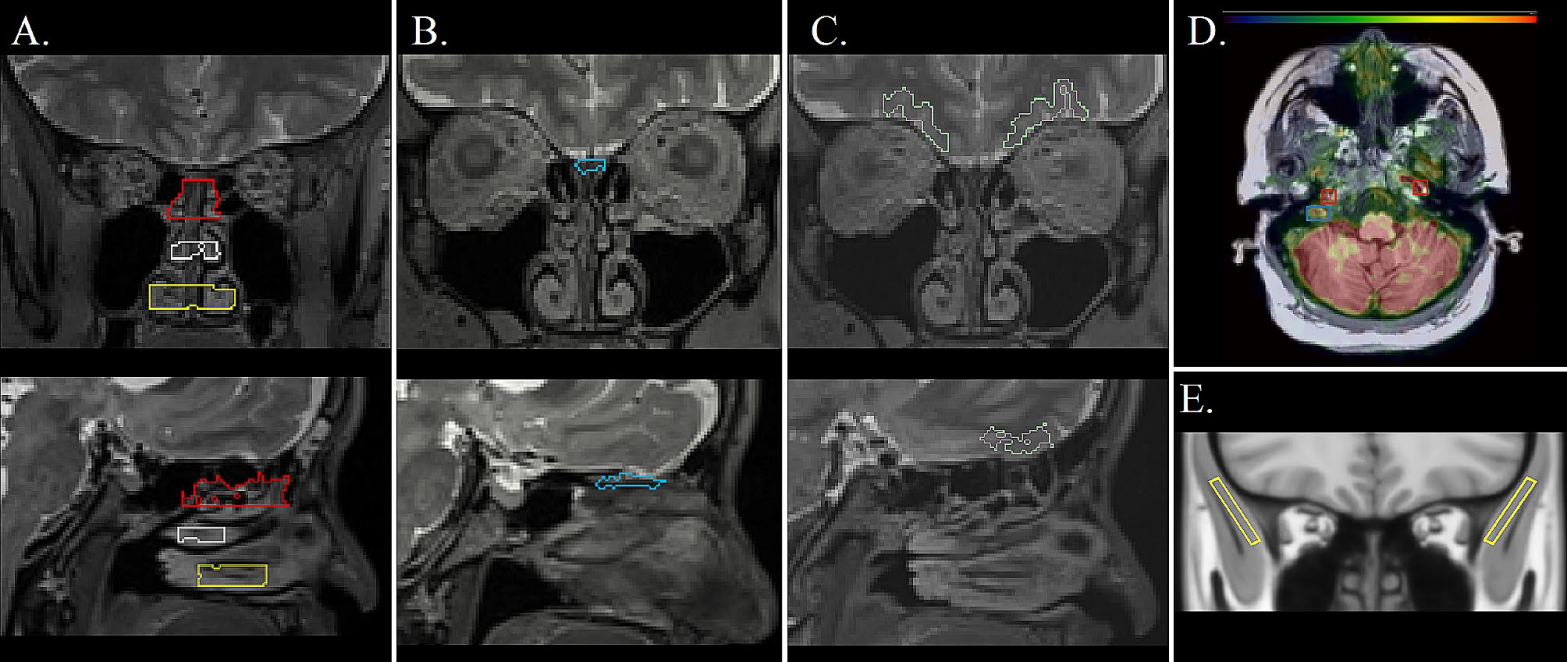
Defining Regions of Interest. Coronal (top row) and sagittal (bottom row) view of ROIs in (A) superior (red), middle (white), inferior (yellow) turbinates displayed on T2W-MRI; (B) the cribriform plate outline (blue) on T2W-MRI; (C) the Freesurfer lateral orbitofrontal (LOF) cortex (green) on T2W-MRI; (D) [1-^11^C]-Butanol PET at 40s post injection displaying on T1W-MRI the location of the seeds for left and right internal carotid artery (red boxes) and right jugular vein (blue box) that were input into vessel-tracking algorithms to generate three-dimensional ROIs; and (E) the temporalis muscle ROI (yellow) was sampled on the MNI152_T1 template
